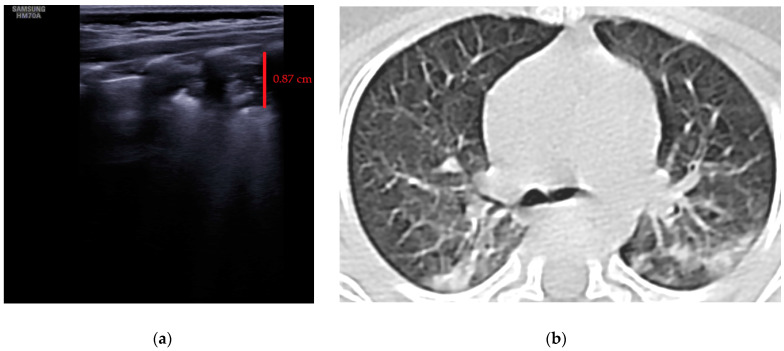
(a) The lung ultrasound showed a small consolidation area with the length <1 cm corresponding to a LUSS = 2; (b) The CT exam revealed bilateral consolidations in the posterior segments.
